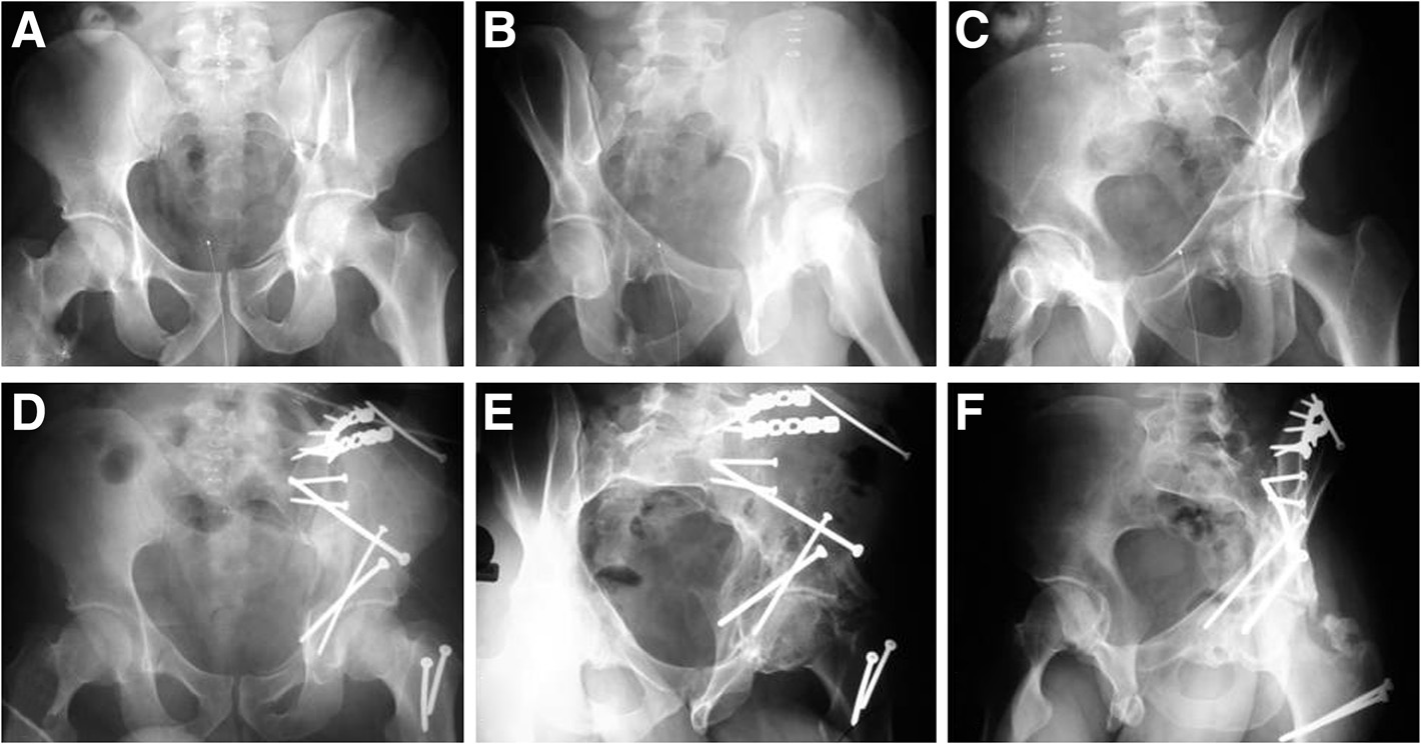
Example of an associated both column fracture treated through an extended iliofemoral approach, with a fracture line extending into the sacroiliac joint. (A-C) Preoperative X-rays (a.p., obturator oblique, iliac oblique). (D-F) Postoperative X-rays (a.p., obturator oblique, iliac oblique).
